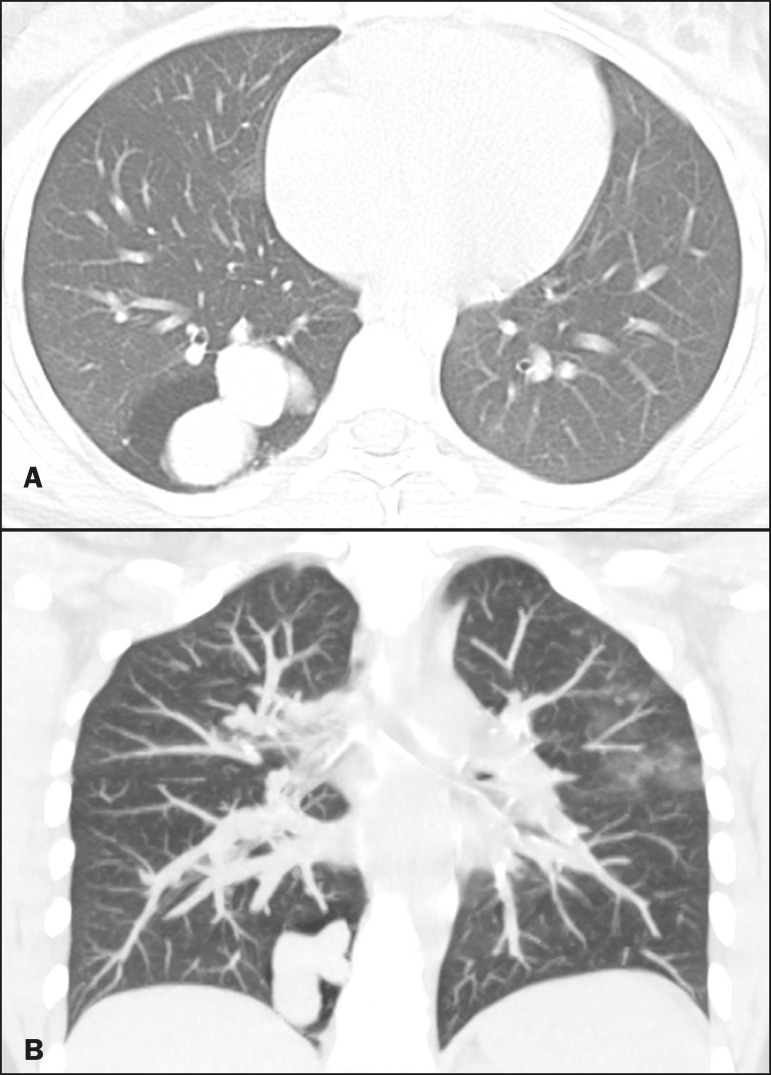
Male, 30-year-old, asymptomatic. Axial CT scan (A), showing nodular opacities (mucoceles) in the right lower lobe, with hyperinflation of the adjacent lung parenchyma. Coronal reconstruction (B), better showing the branching of the mucocele.
